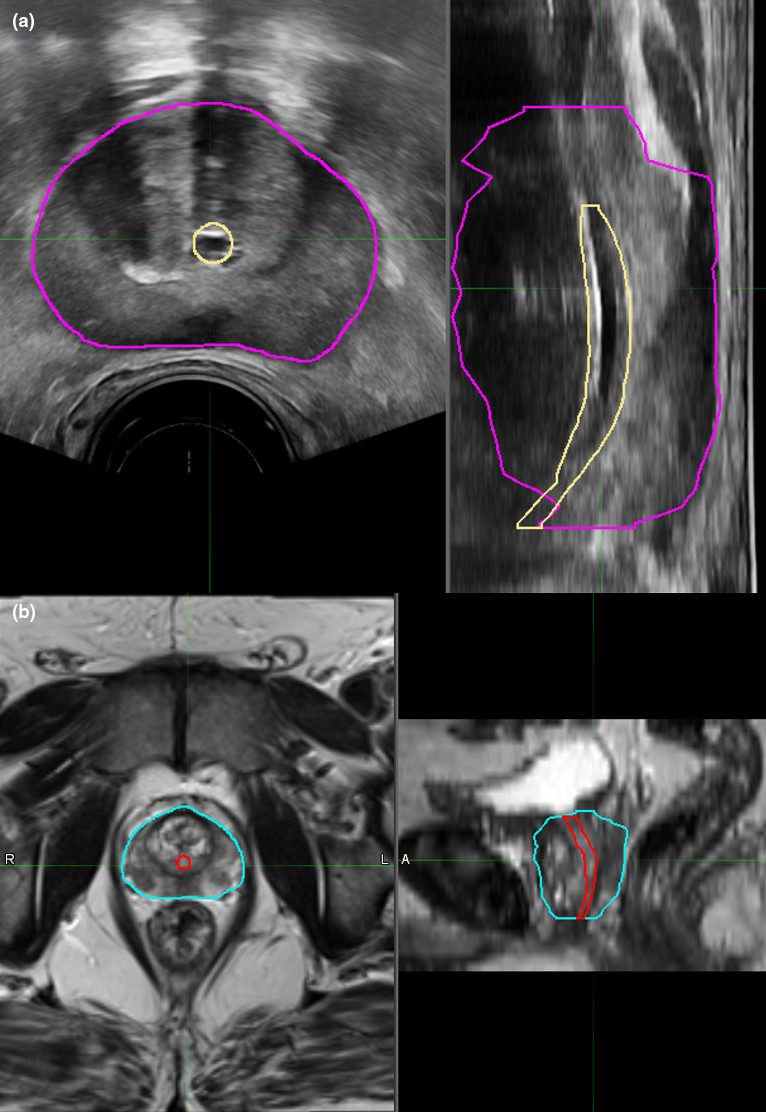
Sample axial and sagittal TRUS (a) and MRI (b) images from this study. The prostate is contoured in purple and cyan and the urethra is contoured in yellow and red on the TRUS and MRI, respectively.
